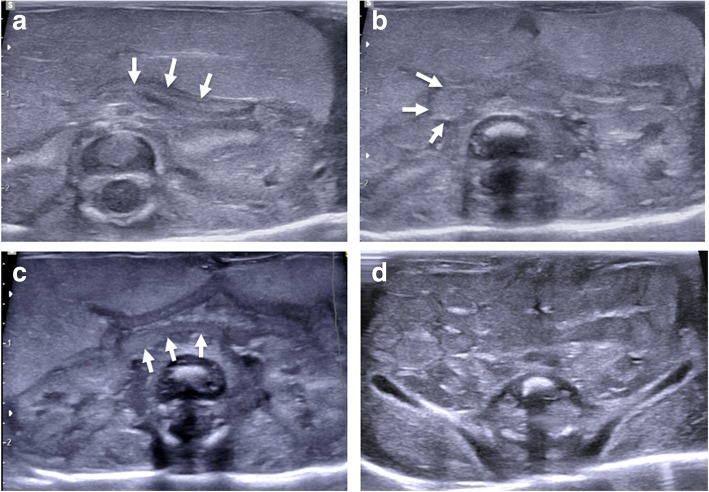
Normal transverse postmortem ultrasound appearances of the bowel in a 19-week gestational-aged foetus, obtained 8 days after death. The first three images (a–c) demonstrate normal rotation of the bowel. In image (a), the gastric pylorus becomes the first part of the duodenum (solid white arrows) and is seen to cross the midline to the right of the abdomen. In image (b), the second part of the duodenum is shown (white arrows), and finally, in image (c), this becomes the third part of the duodenum as it crosses back to the left side of the abdomen (white arrows). In image (d), normal small and large bowel loops in the lower abdomen are seen and meconium filled, without any significant bowel wall thickening or interloop separation
